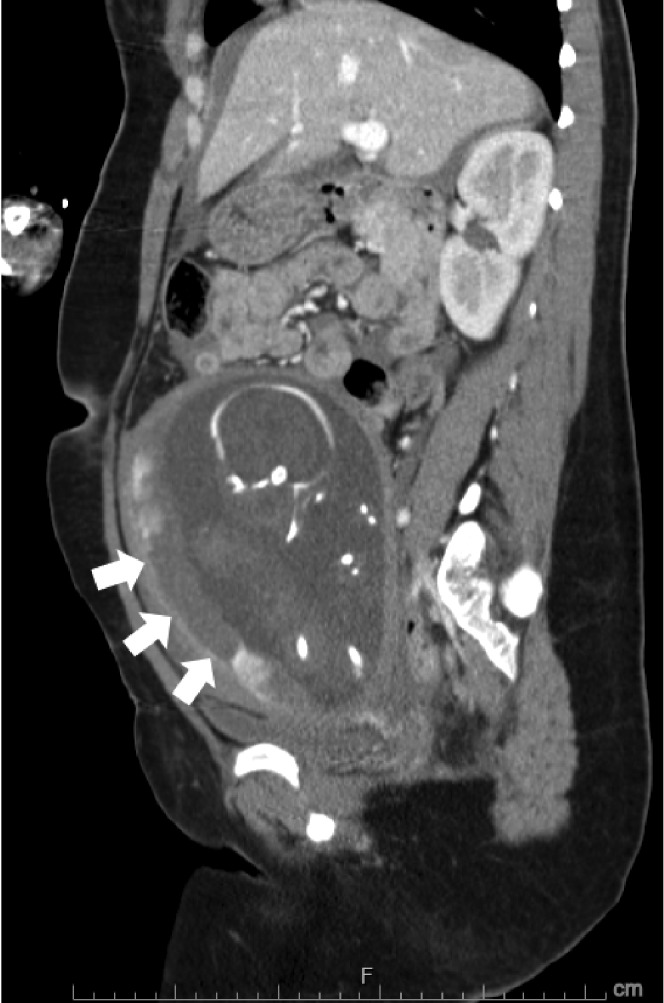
Computed tomography of the abdominopelvic region shows placental damage over the seatbelt-compressed area with a well-circumscribed low-density area (white arrows).
